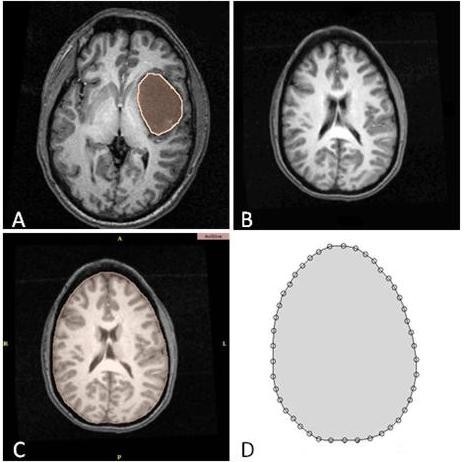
Segmented MRI of the head used to construct a patient specific brain mesh. (a) Tumor segmented in a pre-operative image. (b) Another pre-operative slice of the brain. (c) Brain segmented from image (b). (d) Contour of the brain. Pre-operative images are used to construct computational models of the brain and tumor while the intra-operative images are used to measure the displacements of the anatomical landmarks.
